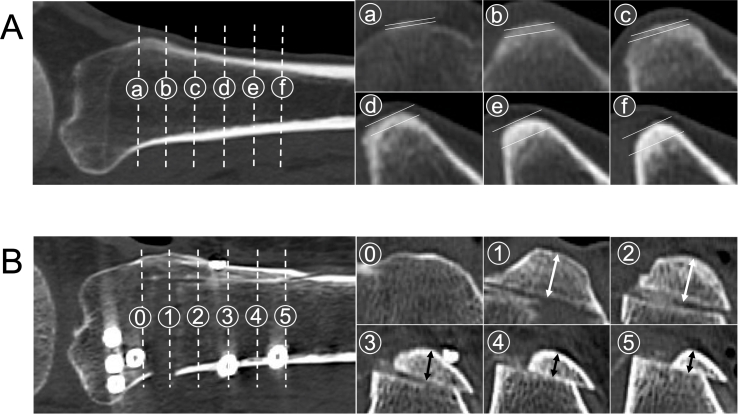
Assessment of the tibial tuberosity. A) Preoperative CT scans of the proximal tibia. ⓐ, ⓑ, ⓒ, ⓓ, ⓔ, and ⓕ indicate 0, 1, 2, 3, 4, and 5 cm from the most proximal part of the tibial tuberosity. The distance between two white lines indicates the thickness of the cortical compartment of the tibial tuberosity. B) CT scans of the proximal tibia 3 days after surgery. ⓪, ①, ②, ③, ④, and ⑤ indicate 0, 1, 2, 3, 4, and 5 cm from the most proximal part of the tibial tuberosity. White or black arrows indicate the thickness of the tibial tuberosity osteotomy.
